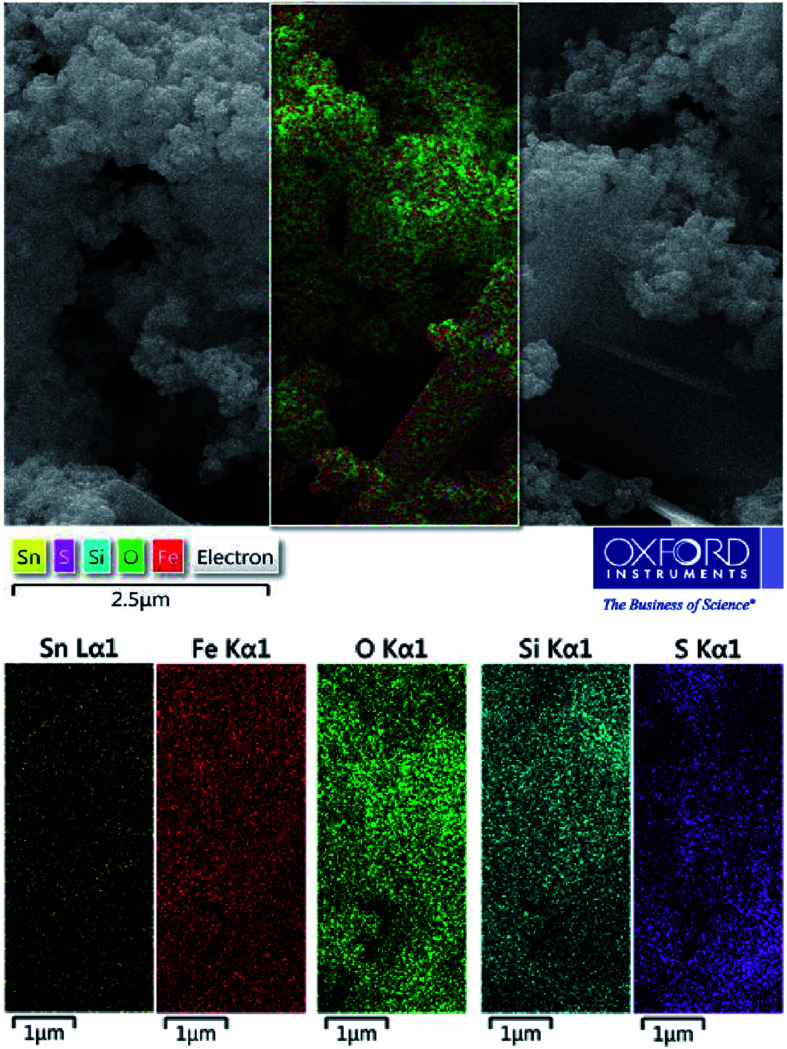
X-ray-mapping analysis of SnFe_2_O_4_@SiO_2_–SO_3_H MNPs.
